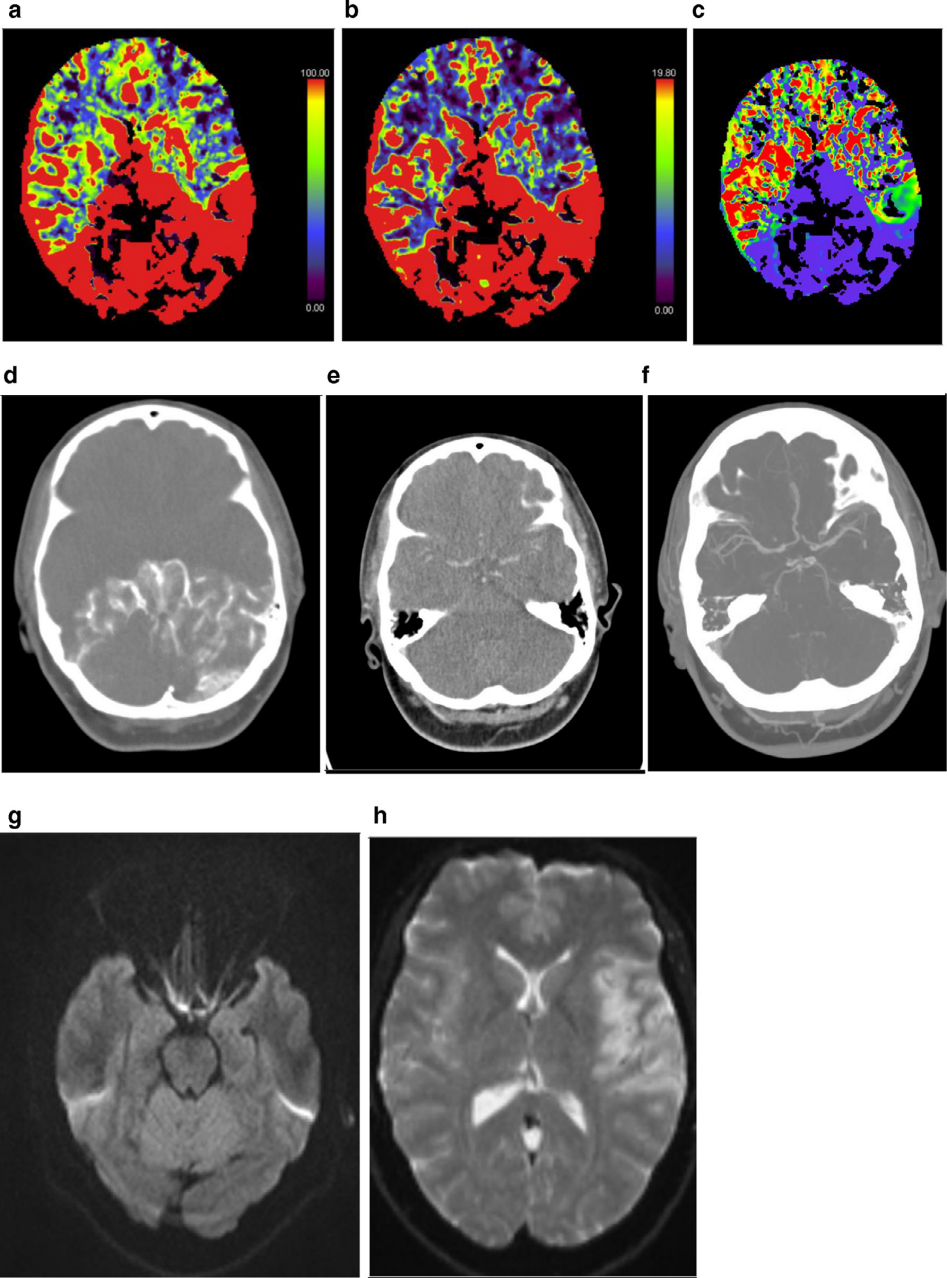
(A) CBF in CT perfusion showing increased flow in the posterior circulation arteries. (B): CBV in CT perfusion showing increased blood volume in the posterior circulation arteries. (C): CT perfusion (MTT) shows decreased transit time in the posterior circulation arteries. (D): CT perfusion early Raw images (VPCT DynMulti4D) – showing increased flow seen in the posterior circulation arteries with absent flow in the anterior circulation in the initial raw images. (E): CT perfusion late raw images showing delayed enhancement of the both anterior and posterior circulation. (F): CT angiography MIP images: Show normal flow in anterior and posterior circulation. (G): MRI, Diffusion weighted images showed no diffusion restriction. (H). MRI, Diffusion weighted images showing diffusion restriction in the left temporo-parietal region.
